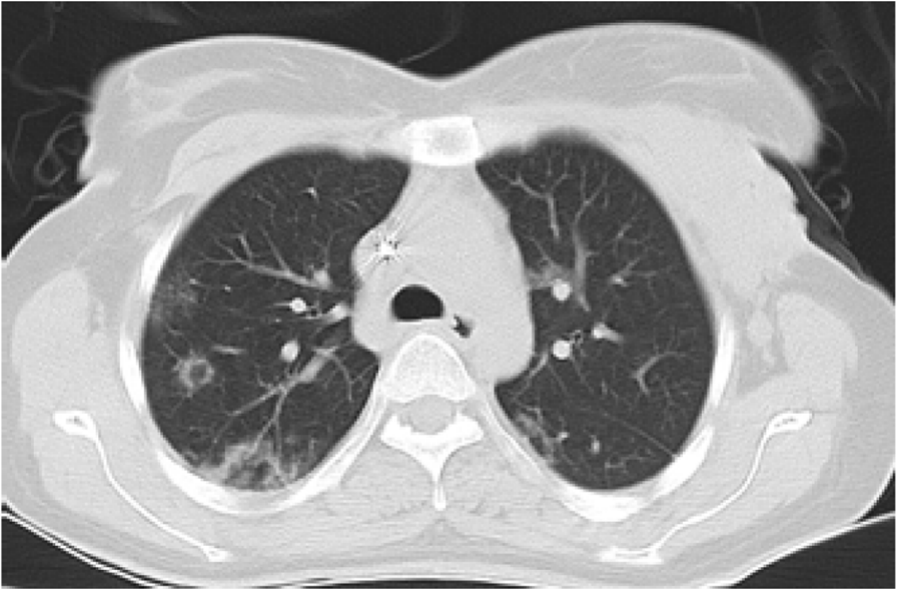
COVID-19 pneumonia: thin section CT shows multifocal peripheral GGO, with reverse halo appearance
